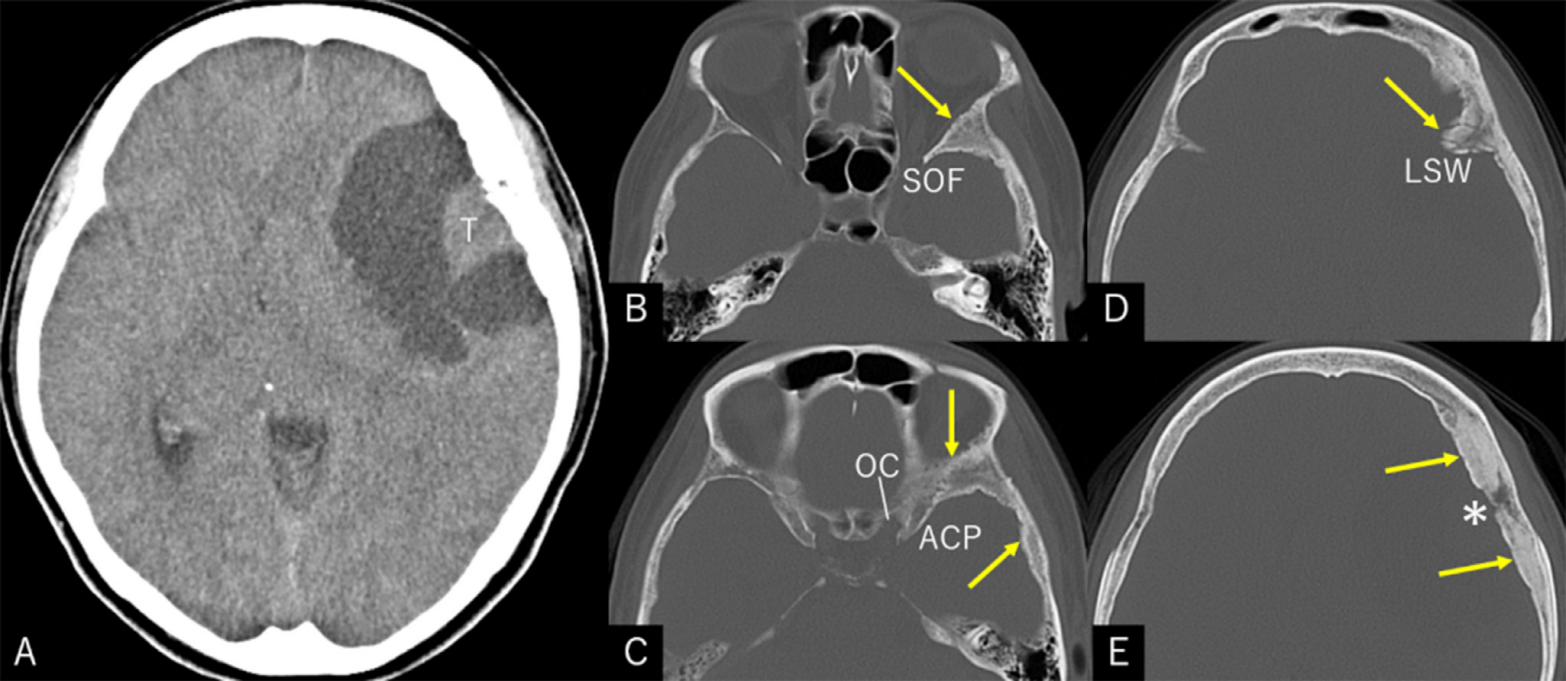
(A) Non-contrast axial computed tomography (CT) scan showing a hypodense cyst in the left frontotemporal region with an isodense tumor included in it. (B–E) Axial bone-target CT scans at the level of the superior orbital fissure (B), anterior clinoid process (C), upper part of the lesser sphenoid wing (D), and the pterion (E) showing extensive hyperostotic changes involving the left sphenoid and frontal bones (*arrows*). The morphologies of the superior orbital fissure and optic canal are intact. *ACP:* anterior clinoid process; *LSW:* lateral part of the lesser sphenoid wing; *OC:* optic canal; *SOF:* superior orbital fissure; *T:* tumor; *Asterisk:* site of tumor attachment.
